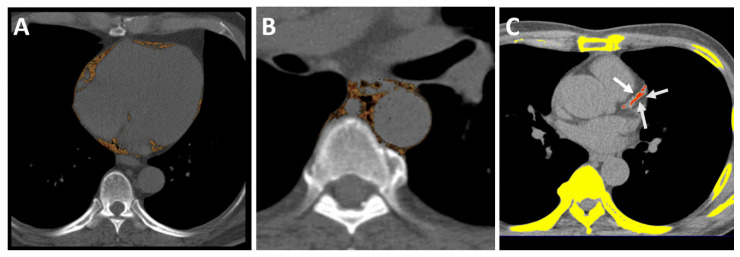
Multidetector computed tomography (MDCT) demonstrated visceral adiposity measures, including pericardial and thoracic peri-aortic fat tissue, and quantification of CACS. The pericardial fat was defined as the fat between the heart and the pericardium, as shown in axial view (A). (A) Pericardial adipose tissue. (B) Thoracic peri-aortic adipose tissue. (C) Semi-automatic quantification of CACS burden using Agatston scoring. *Orange color regions indicate visceral fat tissue. White arrows indicate coronary calcification lesions.
